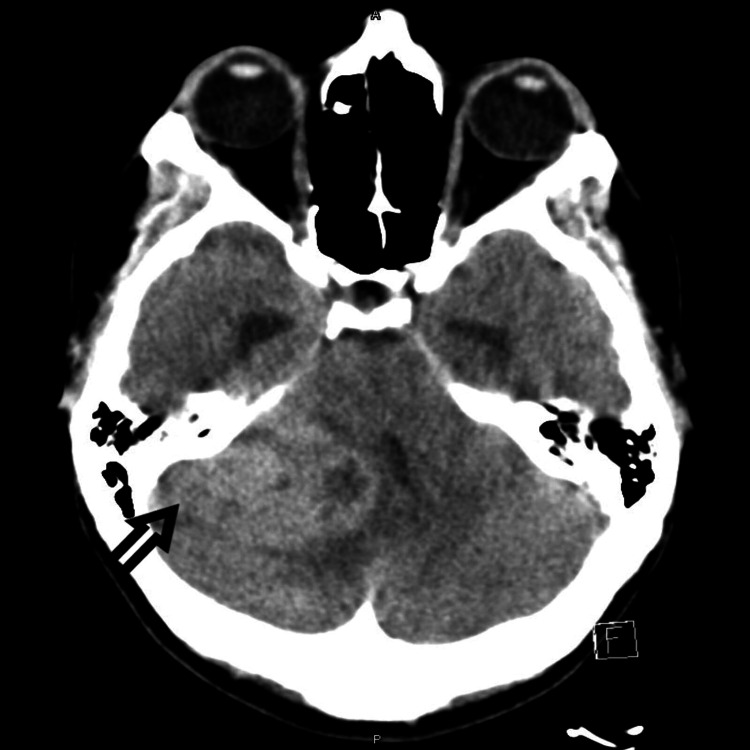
CT scan of the head without intravenous contrast showing a large right cerebellar mass with midline shift in the posterior fossa (shown by arrow).
